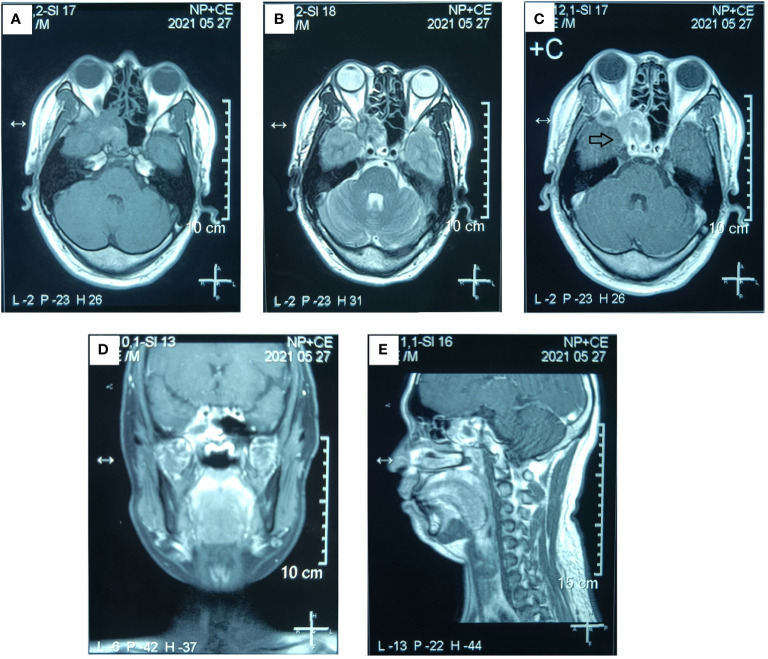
Preoperative MR examination of the paranasal sinus. The MR examination detected a solid mass in the right sphenoid sinus that had extended into the right orbital apex and posterior ethmoid sinus. (A) Axial T1-weighted image. (B) Axial T2-weighted image. (C) Axial T1-weighted enhanced image. (D) Sagittal T1-weighted enhanced image. (E) Coronal T1-weighted enhanced image. (The mass is marked with a black arrow.).
